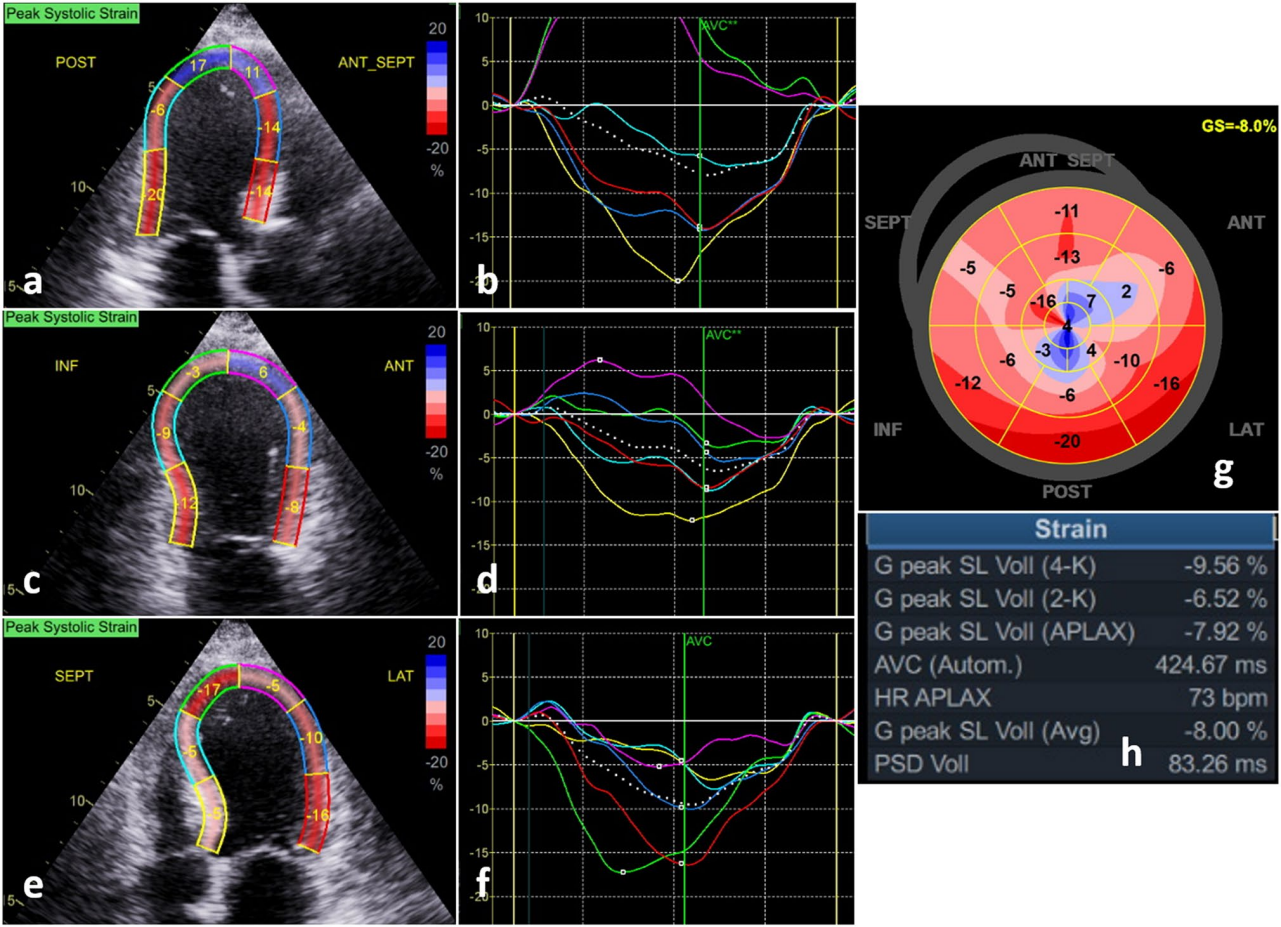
Echocardiographic deformation imaging at acute stage. Peak systolic strain and corresponding regional longitudinal strain curves tracked in the apical long axis view (a, b), the 2-chamber view (c, d) and the 4-chamber view (e, f). The bull’s eye plot of the typical pattern of regional longitudinal strain of the left ventricle is presented in g, the respective strain data are shown in h
